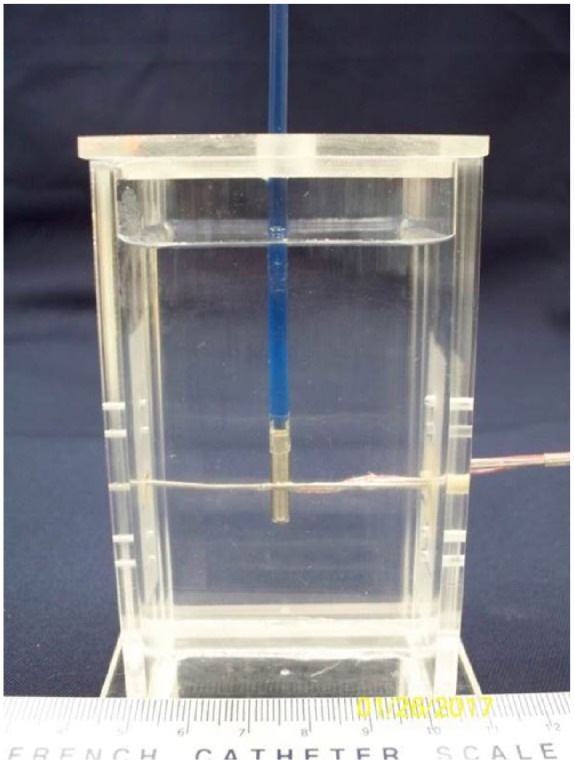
Image of the fully submerged isothermal profile assessment fixture. The fixture contains a water or ultrasound gel medium and a mandrel with thermocouples affixed at five pre-set distances allowing for real-time recording of a freeze run and determination of isotherm location at any point during a given freeze.
